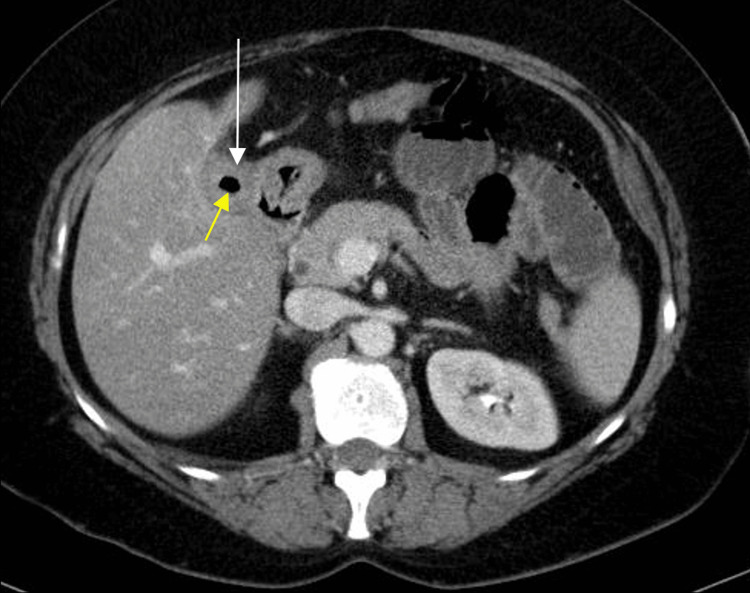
Contrast-enhanced CT abdomen and pelvis through the axial view demonstrating gallbladder wall thickening (white arrow) with gas within the gallbladder lumen (yellow arrow).
